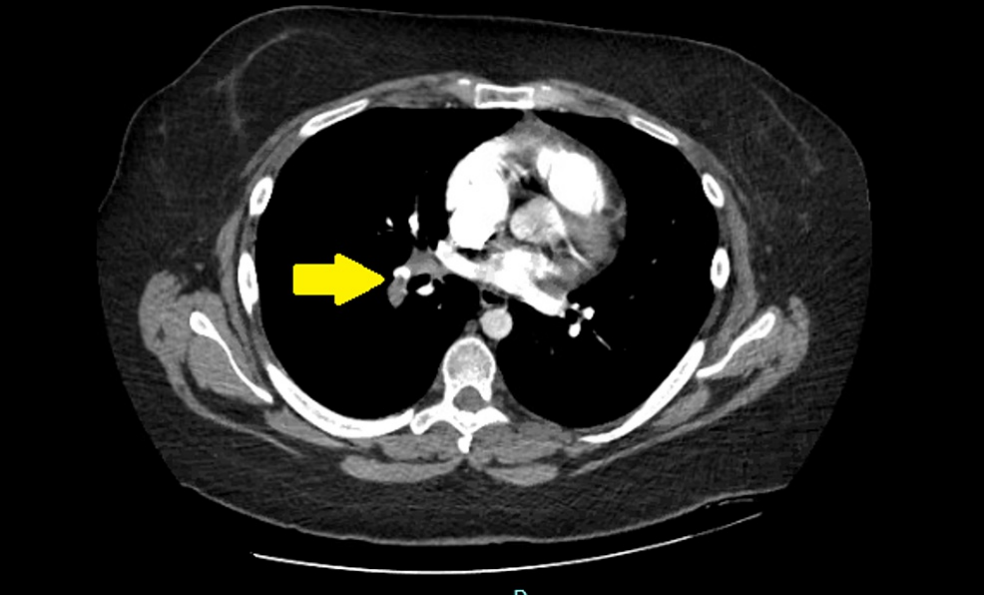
CT of the chest with contrast showing a small right lower lobe pulmonary embolism (yellow arrow). CT: computed tomography
